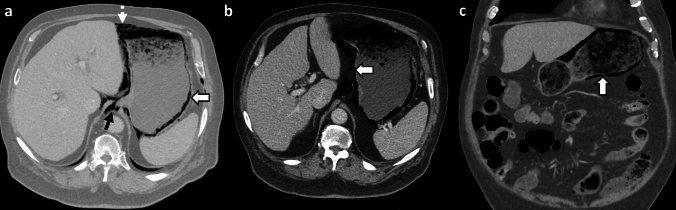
Benign gastric emphysema. A 78-year-old with severe retching, epigastric pain, and Mallory-Weiss tear of the esophagus. CT images show pneumomediastinum at the gastroesophageal junction (black arrow, a), extensive gastric wall emphysema (white block arrow, a–c), and pneumoperitoneum (dotted white arrow, a) tracking down from pneumomediastinum. There is a distinct lack of perigastric inflammation despite extensive gastric emphysema. Serum lactate and WBC counts were normal. The patient was managed conservatively with bowel rest and recovered fully
